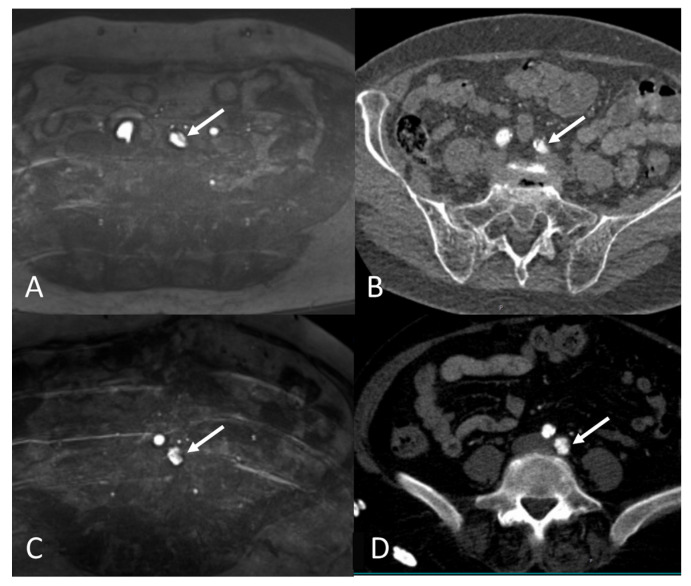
(A,B) MRA (A) and corresponding CTA (B) images of the same patient showing obstructing plaque (white arrow) in left common iliac artery preventing transfemoral access on left side. (C,D) MRA (C) and corresponding CTA (D) images of the same patient showing short dissection (white arrow) of left common iliac artery preventing transfemoral access on left side.
